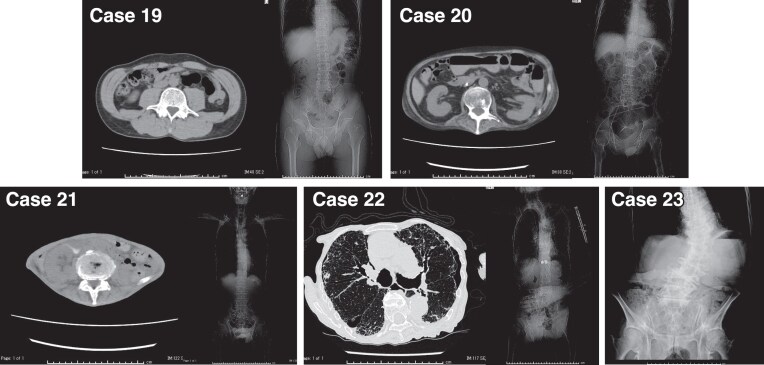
Radiographic images of the patients with major joint pain. Patients 19–22 were thin and patient 22 presented with interstitial pneumonia accompanied by pneumothorax. Patient 23 exhibited scoliosis.
